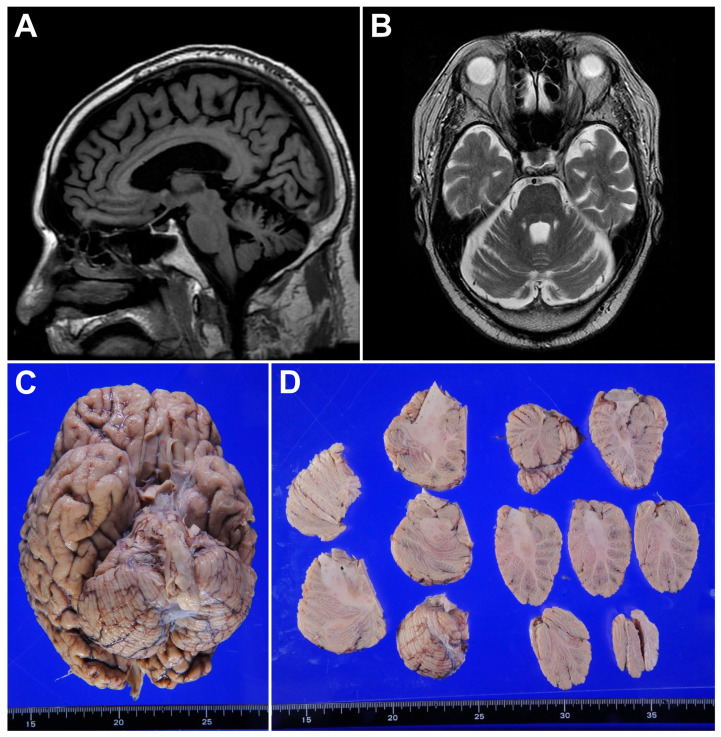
Neuroimaging and gross findings demonstrating mild cerebellar atrophy. (A) T1-weighted sagittal MRI. (B) T2-weighted axial MRI. (C) Basal view of the brain at autopsy. (D) Cut surface of the cerebellum; the right hemisphere was sectioned horizontally, and the left hemisphere was sectioned coronally.
